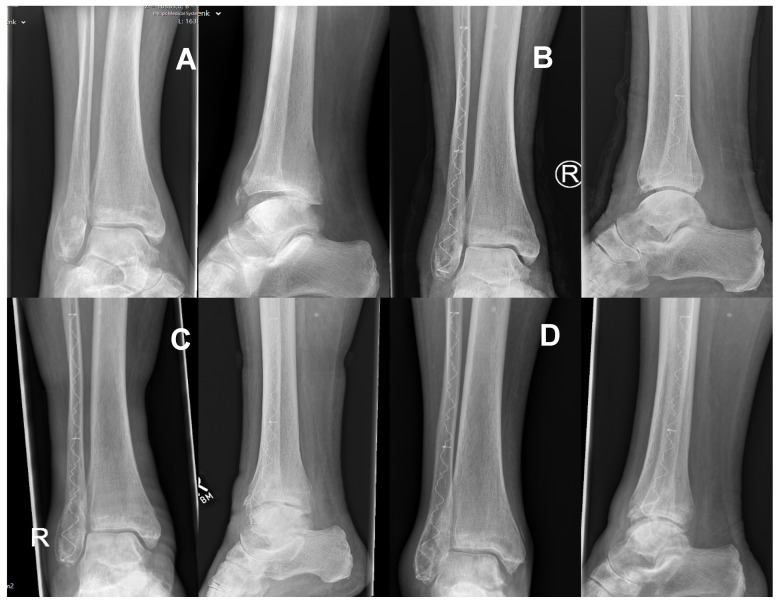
Radiograph of a 77-year-old female patient with an AO type 44 B1.1 ((A) a preoperative) fracture treated with the IlluminOss^®^ Photodynamic Bone Stabilization System. Postoperative results after 6 (B) and 12 weeks (C) as well as after 14 months (D) showed excellent fracture healing.
